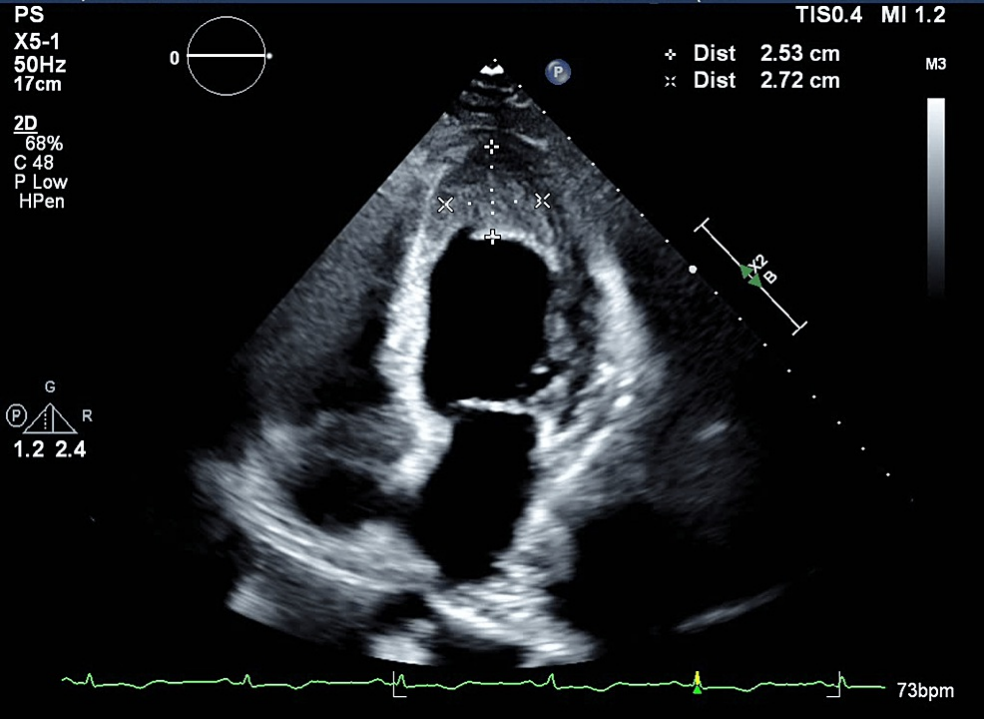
Transthoracic echocardiogram (apical 4-chamber window). Severely hypokinetic apex with a left ventricular ejection fraction of 34% and an associated large, dense, and layered apical thrombus (highlighted in the figure).
